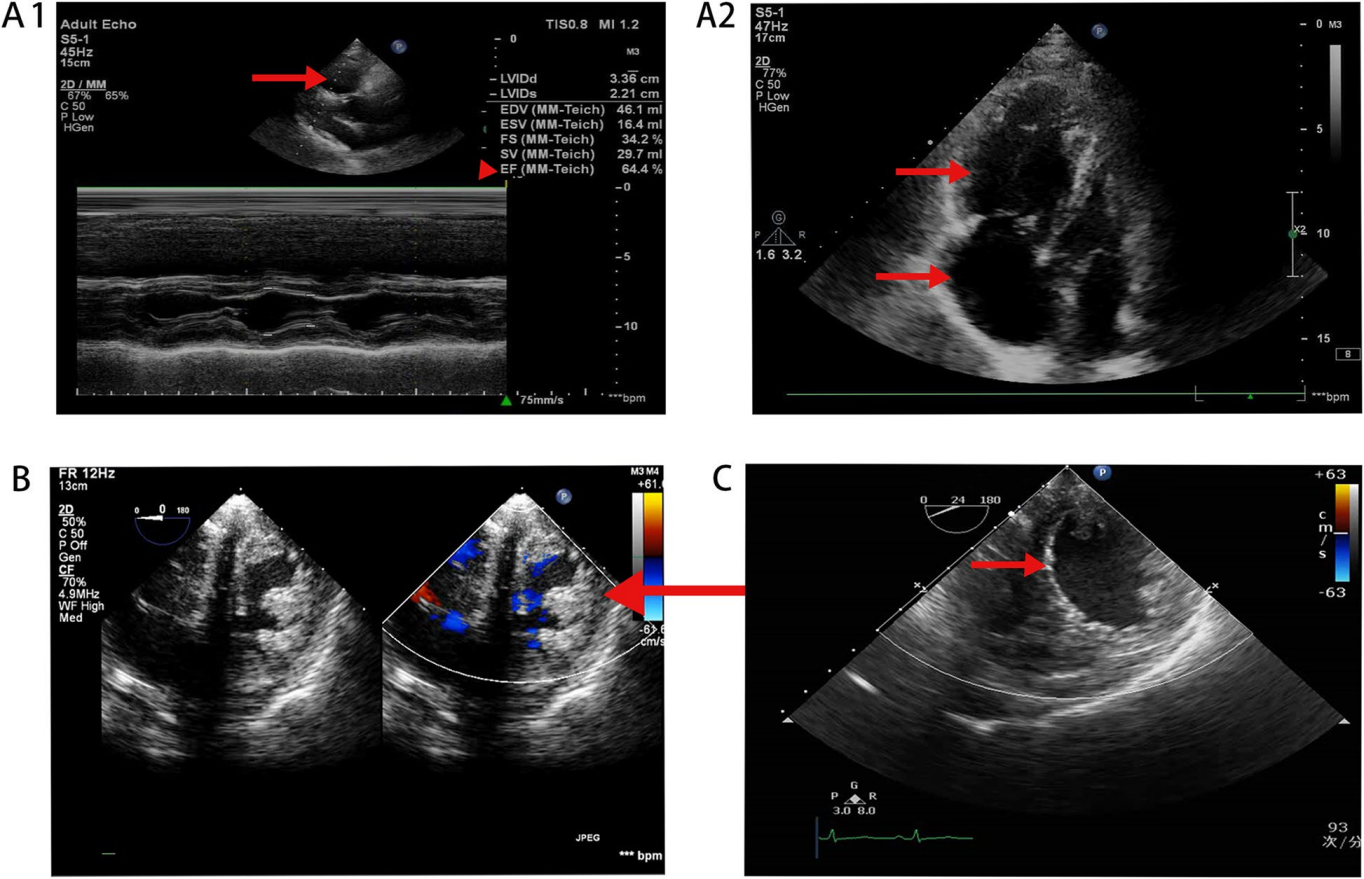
Perioperative echocardiography. Figure 1-A1, A2: Preoperative echocardiography revealed the greatly enlarged right heart with decreased systolic function, and small left heart with normal systolic function (LV34 LA21 RV36 RA49 EF 64.4%). B On POD 1, right heart still dilated significantly with high ventricular pressure, while left heart shrunk obviously and presented like the form of D (as the arrow points to), which indicate left ventricular dysfunction. C On POD 7, echocardiography showed a normal sized heart with satisfying ventricular systolic function. As is shown, right heart had retracted apparently and left heart had recovered to the size of O (as the arrow points to) with improved ventricular function
